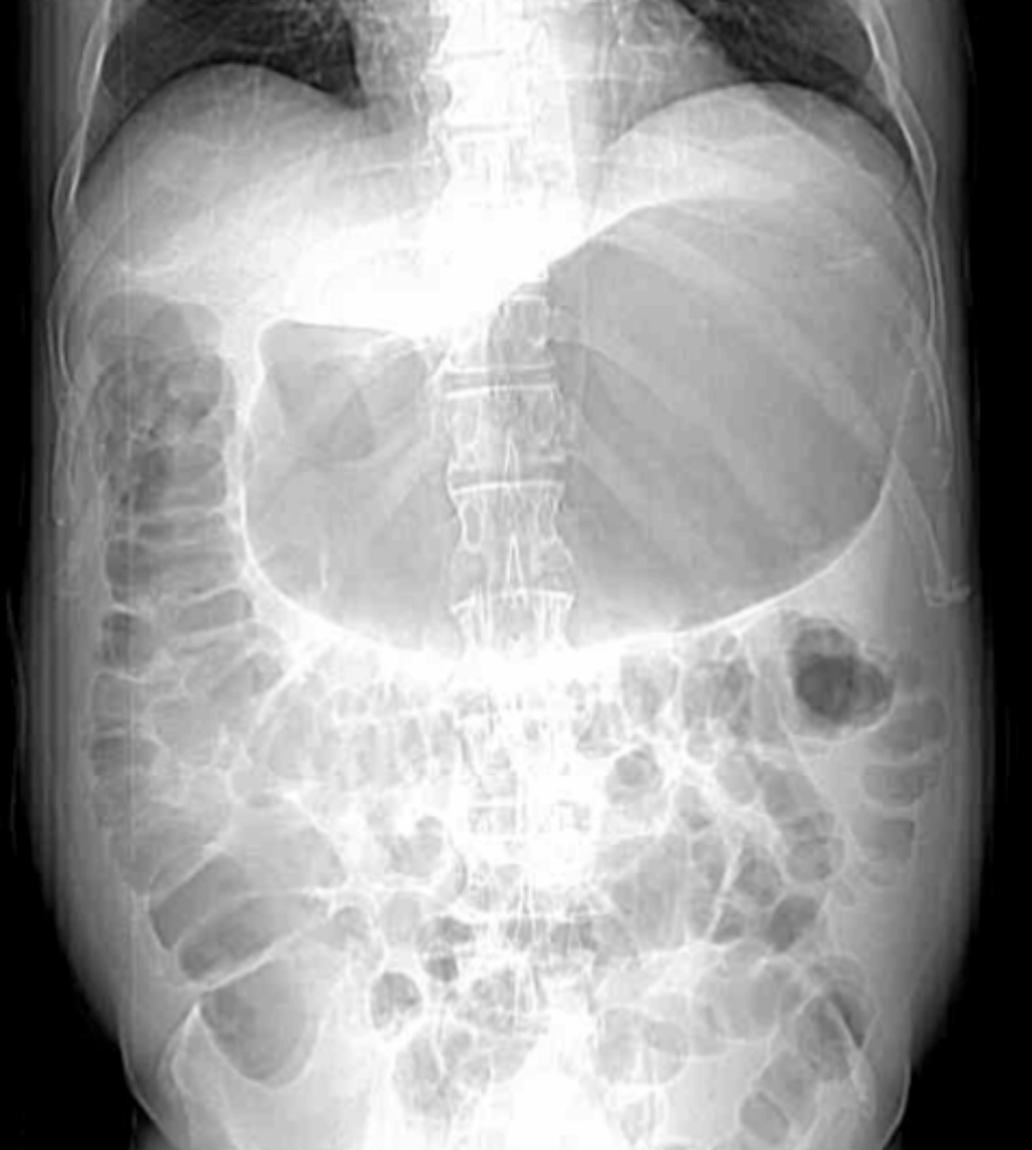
CT of the abdomen and pelvis showing markedly distended stomach
